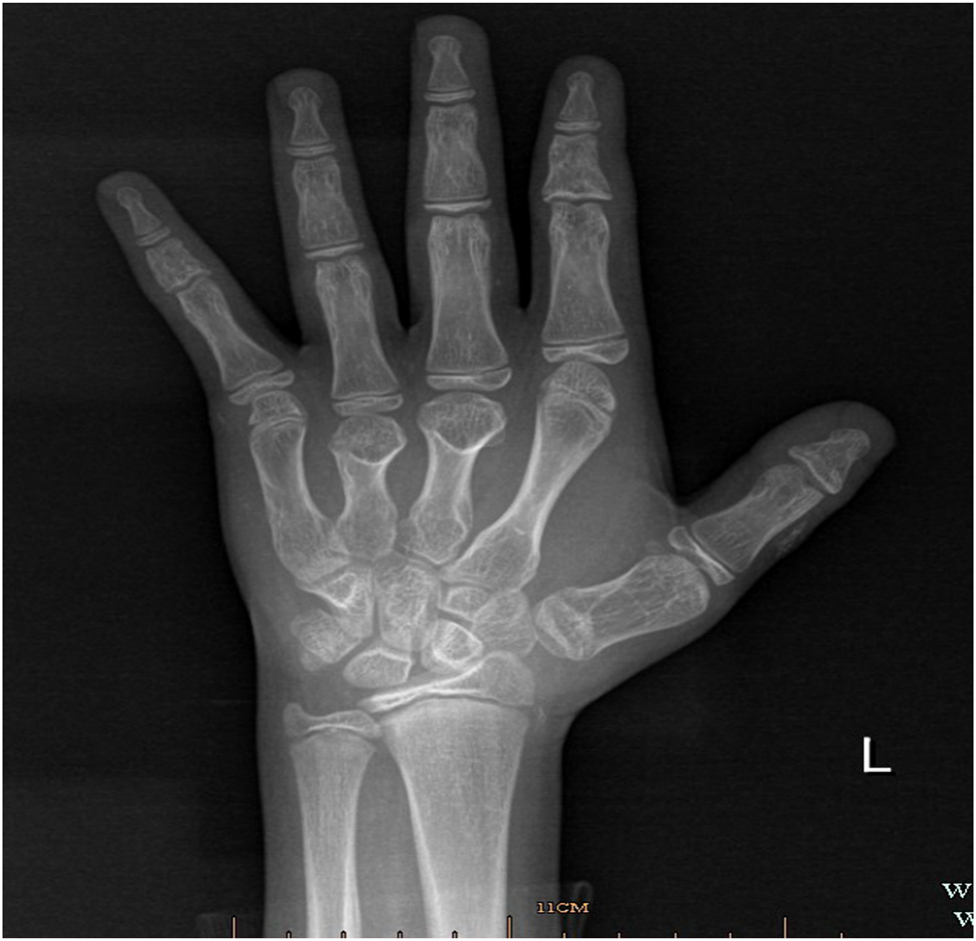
The left-hand X-ray result of the patient.
